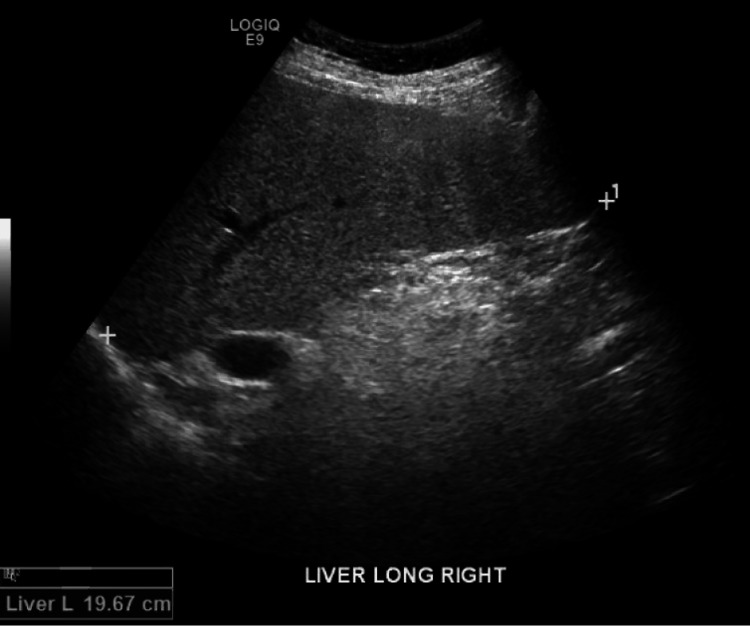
Hepatomegaly found on patient’s abdominal ultrasound
